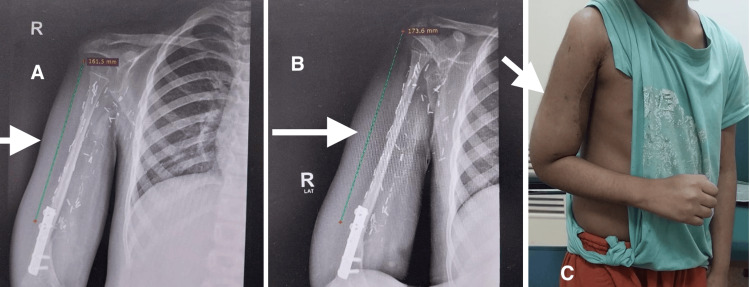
X-rays and cinical image of the patient at the 12-month follow-up (A) X-ray (anterioposterior view) showing adequate fixation; (B) X-ray (lateral view); (C) Patient's reconstructed limb after 12 months
